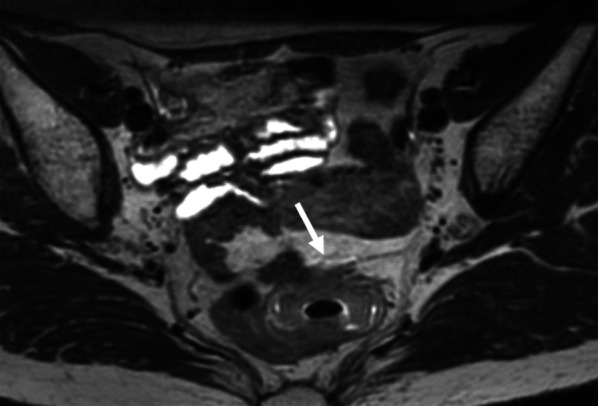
Preoperative magnetic resonance imaging (MRI). MRI revealed the tumor had invaded beyond the muscular layer of the rectum (arrow)
